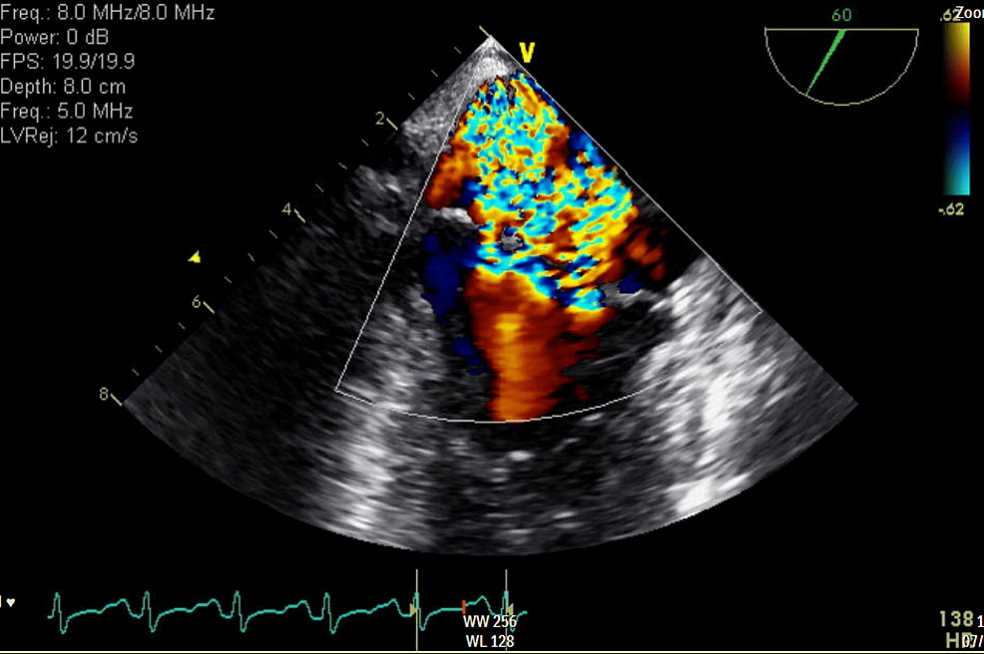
Transthoracic echocardiogram showing trace mitral regurgitation at the end of systole​
